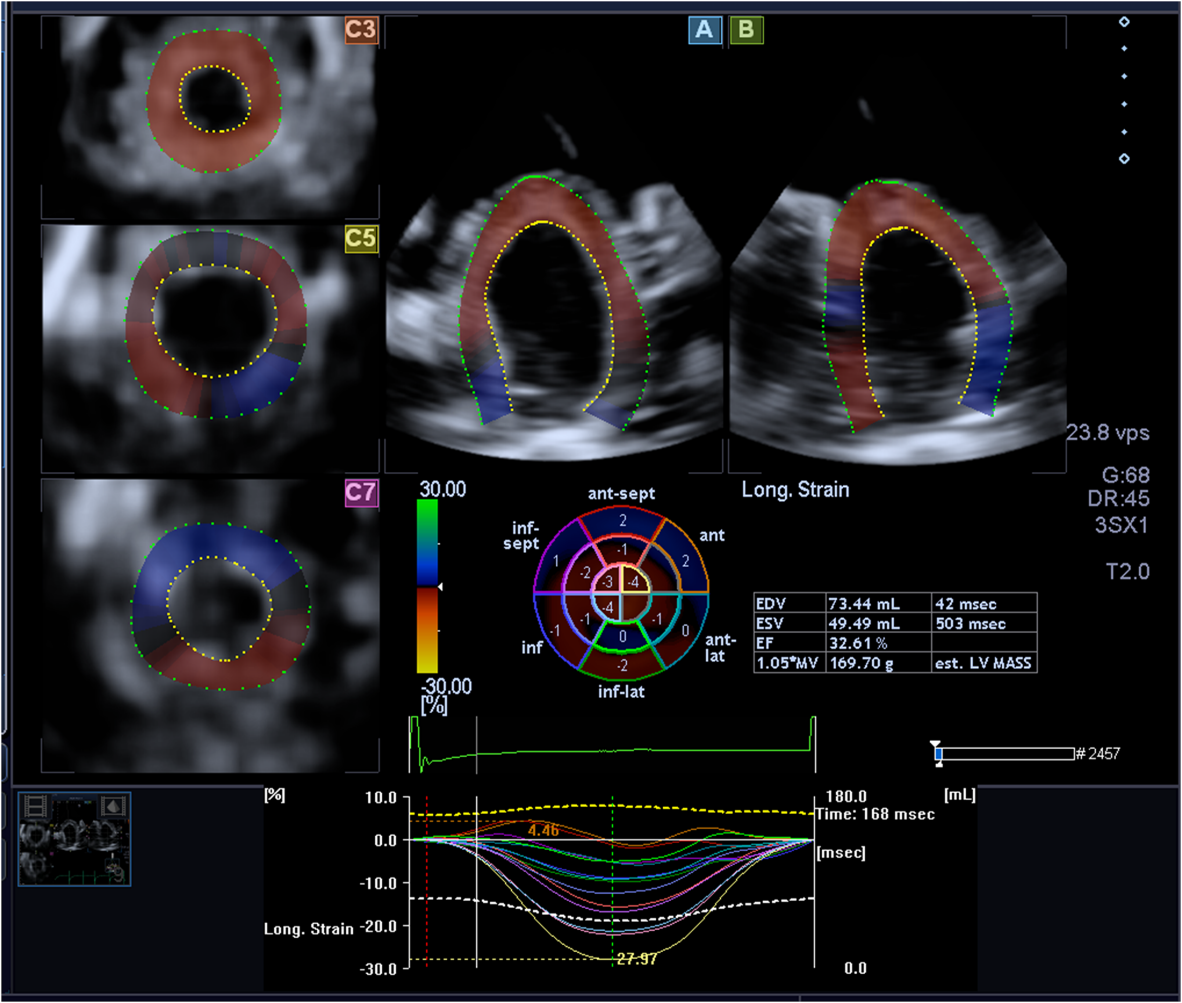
Strain Values derived by three-dimensional speckle tracking program: 3D datasets were displayed in 2 cross-sectional planes. Panel A and B showed longitudinal apical four-chamber and two-chamber views displayed on the right side. 3 short-axis views were displayed on the left side, which were redefined by adjusting the lines in both apical longitudinal views at different short-axis levels (apex, middle, base) to get clear cardiac borders. The region of interest was defined by points at the right and left mitral annulus as well as the apex at end-diastole in apical four-chamber and two-chamber views; the program automatically generated endocardial and epicardial border lines which were manually adjusted in all long-axis planes and short-axis planes. 3D strain parameters could be directly acquired in an exported Excel spreadsheet.
